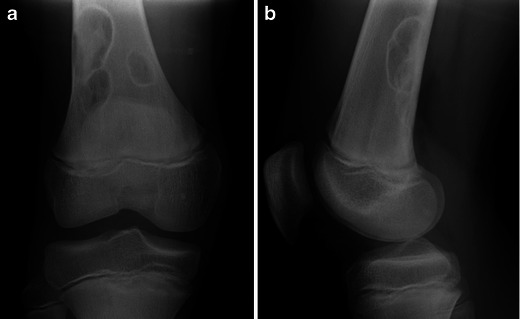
Frontal (a) and lateral (b) X-rays of the right knee: Non-ossifying fibroma—typical radiological aspect
